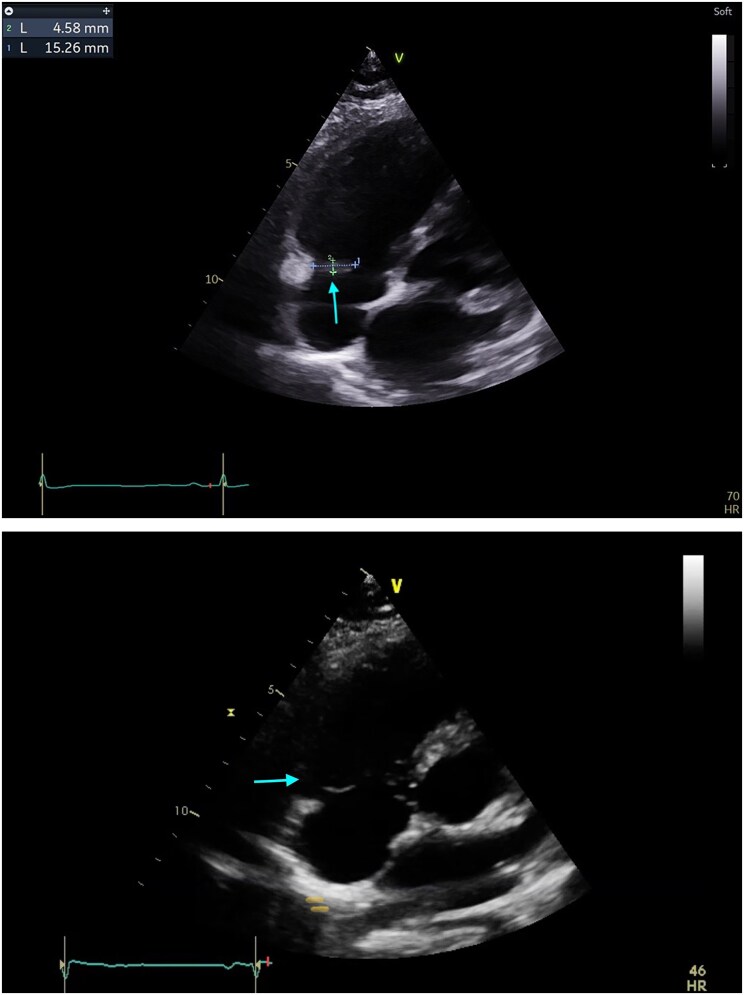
(*A*) Follow-up transthoracic echocardiography performed by the same operator after 1 week of anticoagulant at Day 21 on the Timeline revealed a persistence of one lobe (15 mm × 5 mm) pediculated mobile masse attached to the atrium side of the lateral tricuspid annulus (see arrows). One lobe of masse disappeared compared to the first transthoracic echocardiography (*Figure 2*). (*B*) Follow-up transthoracic echocardiography at Day 27 from initial presentation (18 March 2025), 4 days after removal of port-a-cath (14 March 2025), showed a complete resolution of the mass at the lateral side of the tricuspid annulus (see arrows).
